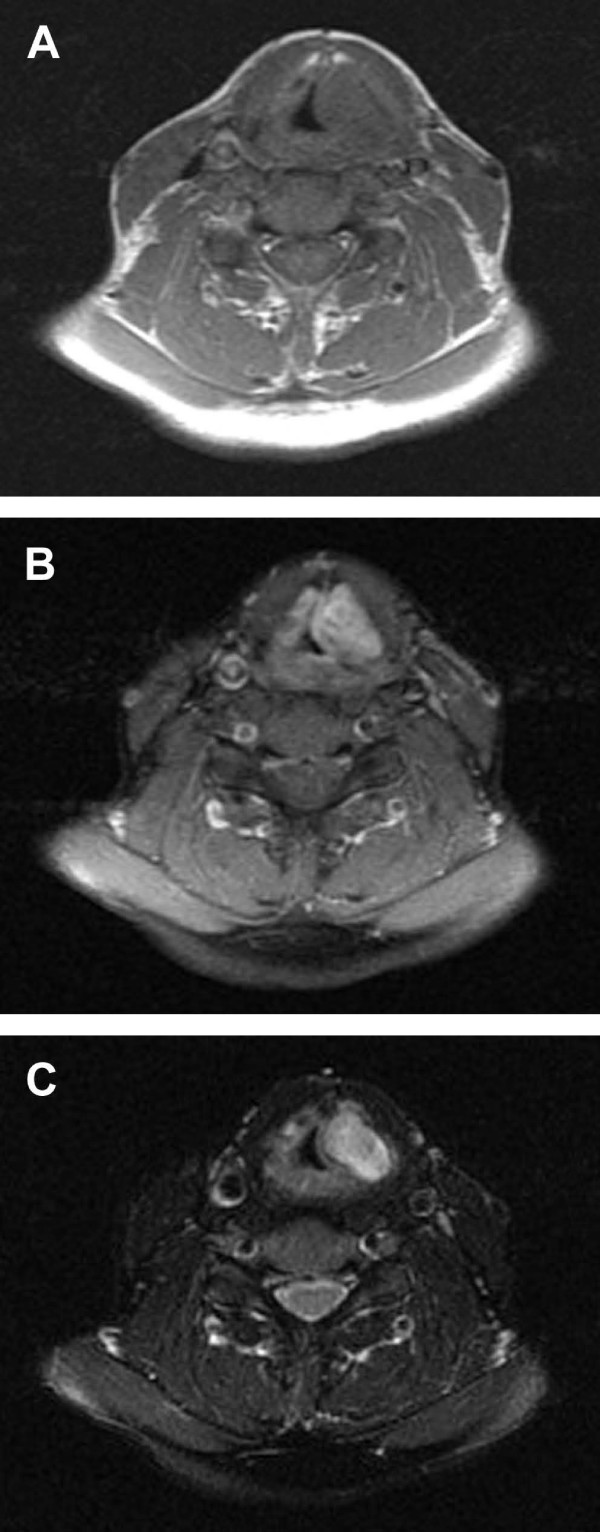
MRI images of the supraglottic tumor. (A) T1-weighted image without Gadolinium, (B) T1-weighted image with Gadolinium, (C) T2-weighted image.
